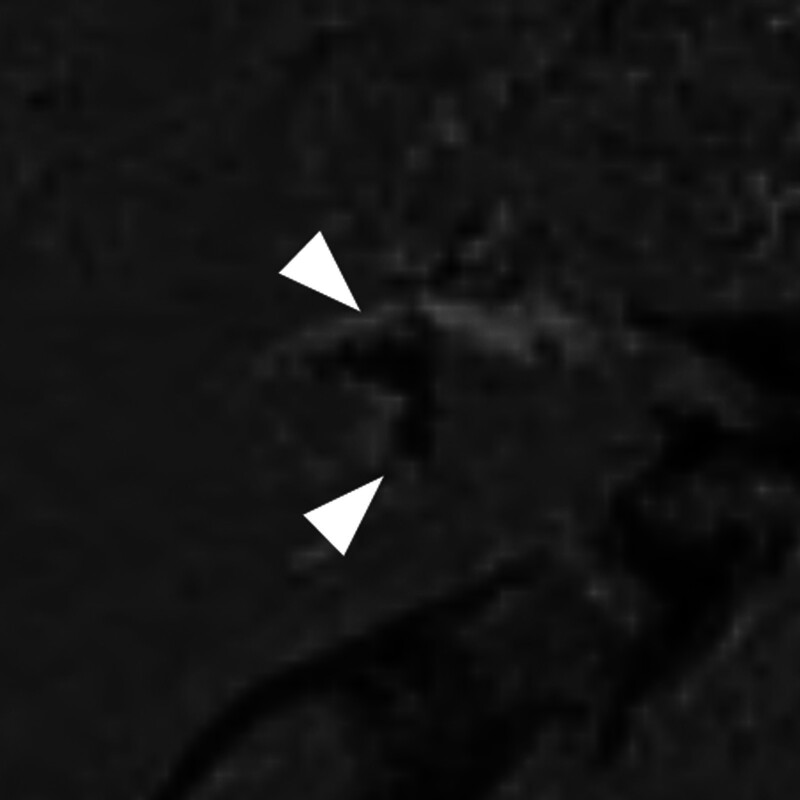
Inner ear magnetic resonance imaging (MRI) of a 46-year-old female with dizziness and right ear fullness. Grade II vestibular hydrops is detected on a 0.1 mm axial hybrid of reversed image of positive endolymph signal and native image of positive perilymph signal (HYDROPS) image of inner ear MRI at vestibular level, in which a complete confluence of saccule and utricle (arrowheads) with enhancement of the perilymphatic rim is visible.
